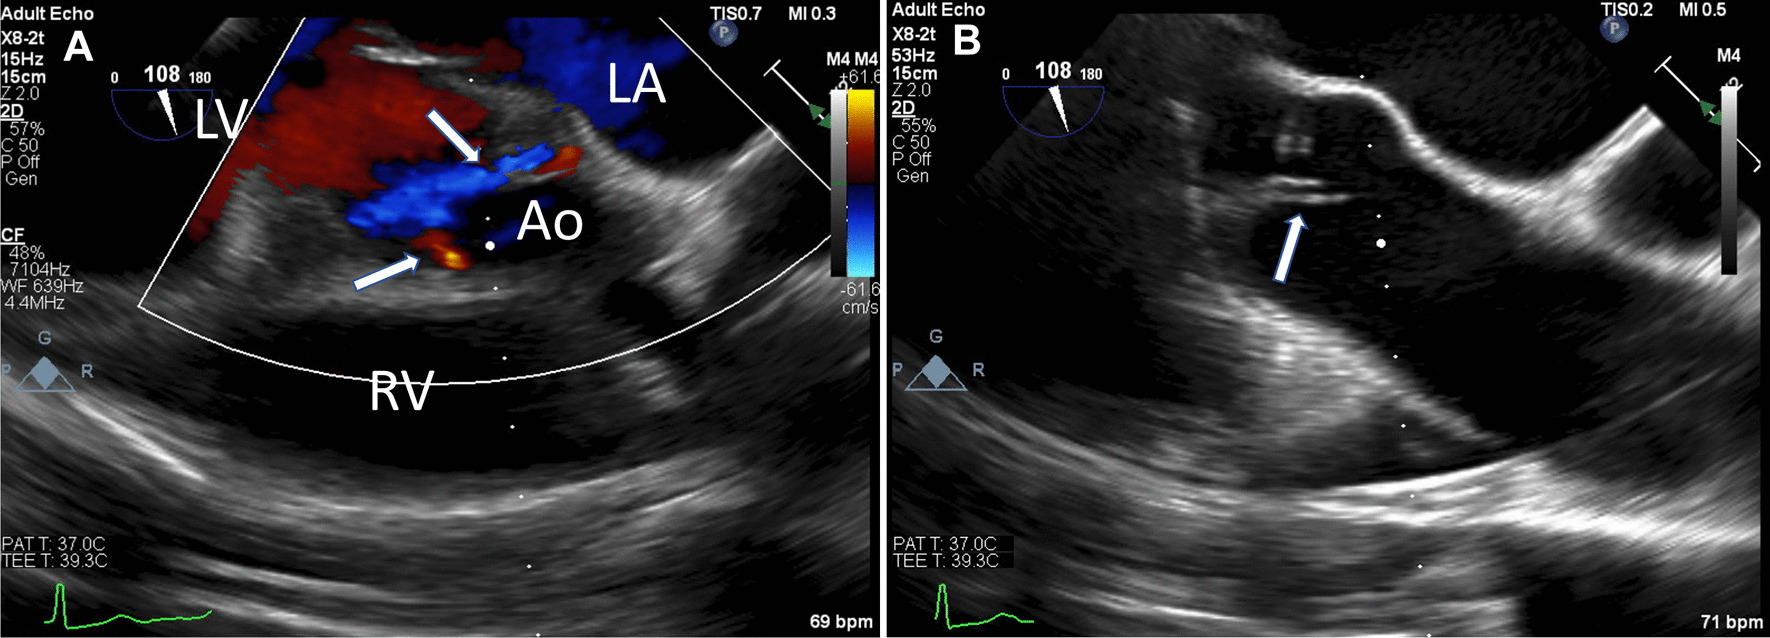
A Mid-esophageal transesophageal echocardiogram image displaying two regurgitant jets (arrows) flowing from the aorta (Ao) to the left ventricle (LV), indicative of new onset mild-to-moderate aortic regurgitation; RV, right ventricle; LA, left atrium. B A structurally normal aortic valve (arrow) observed in the same view using standard ultrasound mode
